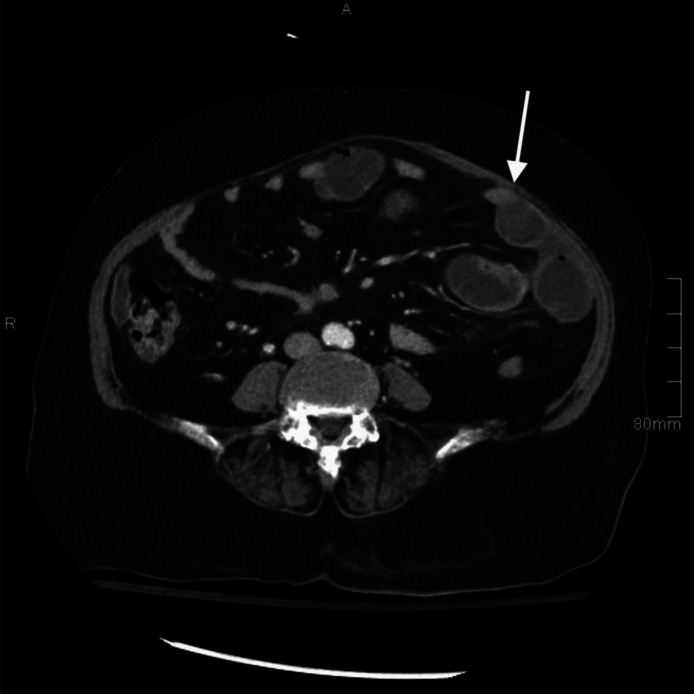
Axial contrast-enhanced CT image. The image shows a dilated mid-jejunal loop with a maximum diameter of 3.2 cm. A transition zone was observed at the distal jejunal loops (white arrow). Minimal pericholecystic-free fluid was noted. The overall findings raised the suspension of a radiolucent gallstone, eroded through a cholecysto-enteric fistula due to underlying chronic cholecystitis, with subsequent jejunal loop obstruction.
